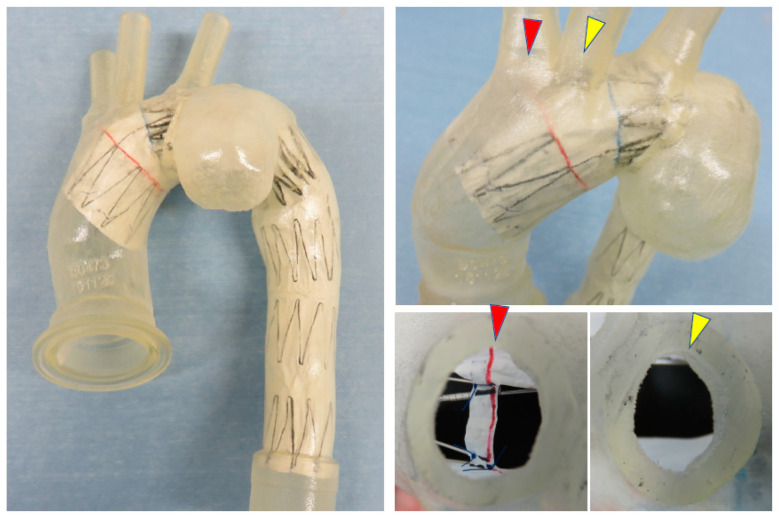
Using CT data, a 3D-printed vascular model of a patient is fabricated, allowing for the placement of the prototype Najuta to assess its conformance and alignment with the fenestrations for the brachiocephalic artery (red arrows) and the left common carotid artery (yellow arrows).
